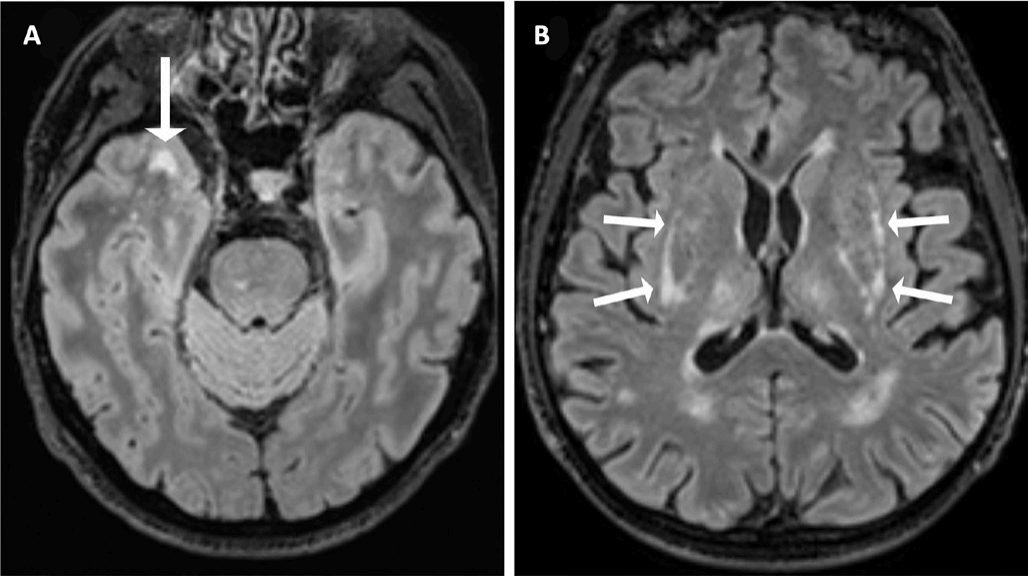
Axial T2 FLAIR brain MRI of two subjects with a pathogenic *NOTCH3* variant in UK Biobank. (A) A woman in her 50s, carrying a p.Arg1231Cys variant that affects EGFR domain 31, with WMH involving the right anterior temporal lobe (arrow). (B) A man in his 60s, carrying a p.Arg578Cys variant that affects EGFR domain 14, with WMH involving the external capsule bilaterally (arrows). EGFR, epidermal growth factor-like repeat; FLAIR, fluid attenuated inversion recovery; WMH, white matter hyperintensity.
